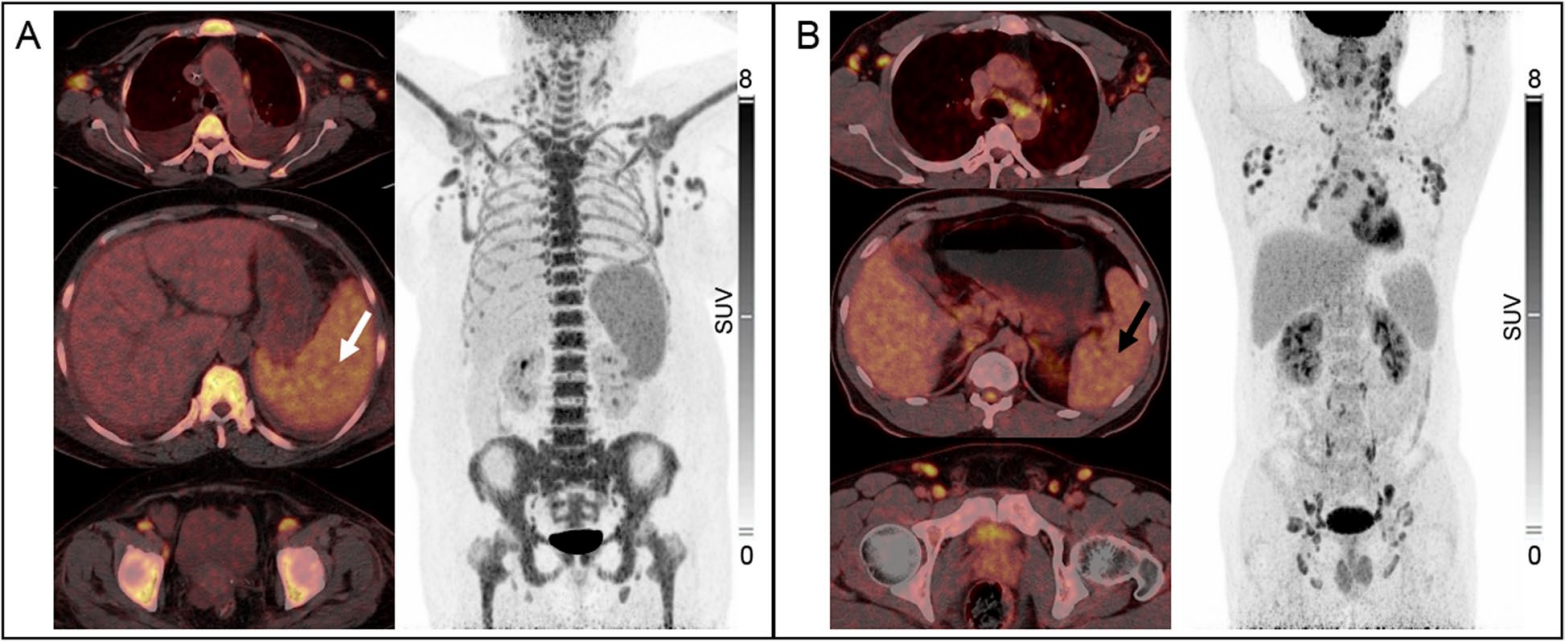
A High severity case: A 67-years-old female, admitted to the hospital with asthenia, abdominal pain and diarrhea. She was finally diagnosed as plasma cell type iMCD, disease severity grade 4 (as per CTCAE 5.0 standards), and the iMCD risk stratification was severe (as per CDCN standards). PET/CT showed multiple serous cavity effusion, multiple enlarged lymph nodes in the whole body with increased FDG uptake, diffused increased metabolism in the bone marrow and increased metabolism in the spleen (SUV_max_ 5.03, as indicated by the white arrow), and the SLR was 1.99 (SLR > 1.04). B Low severity case: A 33-year-old male was admitted to the hospital due to abnormal renal function and lower limb edema. He was ultimately diagnosed with hyaline-vascular type iMCD, with a CTCAE grade of 0, and the risk stratification for iMCD was non-severe. PET/CT revealed multiple enlarged lymph nodes throughout the body accompanied by increased FDG uptake. the spleen showed no high metabolism (SUV_max_ 3.3, as indicated by the black arrow), and the SLR was 0.83 (SLR < 1.04)
